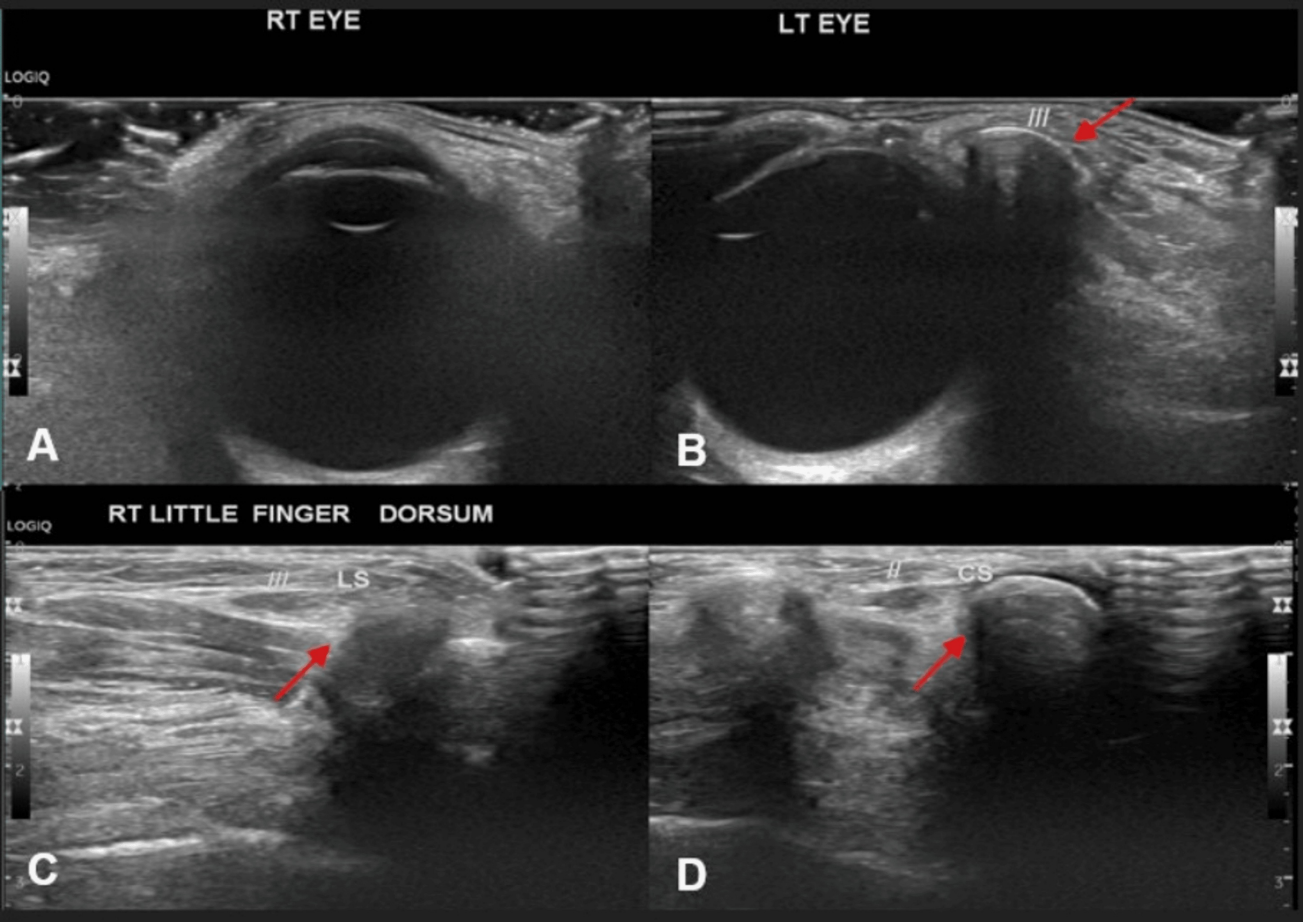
Ultrasound of the left eye and right little finger showing orbital and adnexal cysticercosis Ultrasound image of normal right eye (A) for comparison. Ultrasound image of the left eye (B) showing a well-defined oval hypoechoic area (indicated by red arrow) measuring ~10 x 6 mm seen in the subcutaneous plane of the left orbit - likely uncalcified cysticercosis. Ultrasound images of the dorsum of the right little finger in longitudinal section (C) and cross-section (D) showing a well-defined oval hypoechoic area in the muscular plane (indicated by red arrows) - likely uncalcified cysticercosis.
